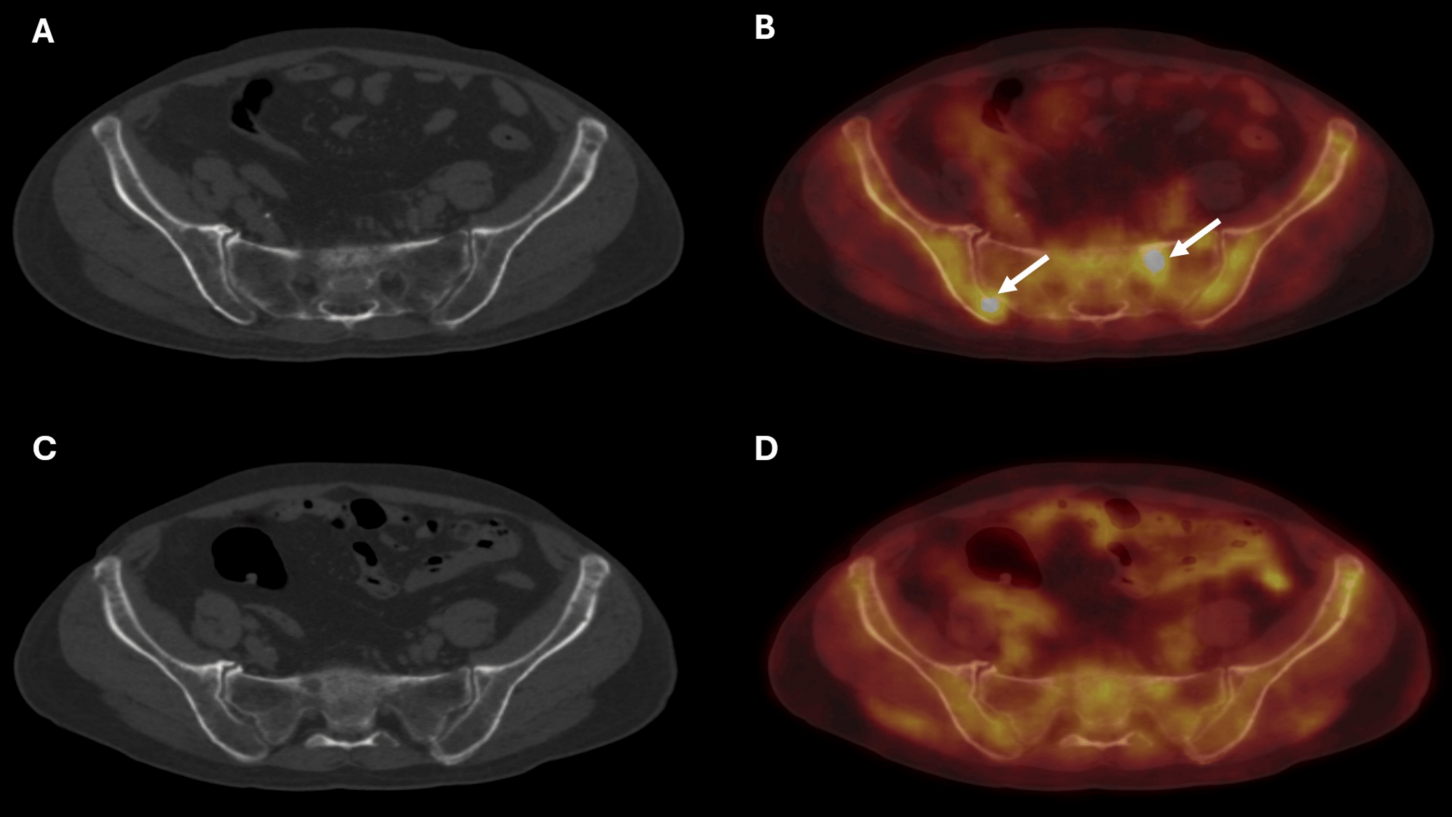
68Ga-CXCR4 and 18F-FDG PET/CT axial images of the patient (A) Axial view of CT. (B) Axial view of 68Ga-CXCR4 PET/CT fusion. (C) Axial view of CT. (D) Axial view of 18F-FDG PET/CT fusion. Image B shows increased 68Ga-CXCR4 uptake in the left ala of the sacrum and right iliac bone (arrows). Image D reveals no focal FDG uptake in the left ala of the sacrum and right iliac bone. Images B and D also show diffuse increased radiotracer uptake involving bilateral iliac bones and sacrum for both the radiotracers. In this example, CXCR4 PET/CT identifies more areas of focal radiotracer uptake CXCR4: C-X-C chemokine receptor type 4; 18F-fluorodeoxyglucose; PET/CT: positron emission tomography/computed tomography
